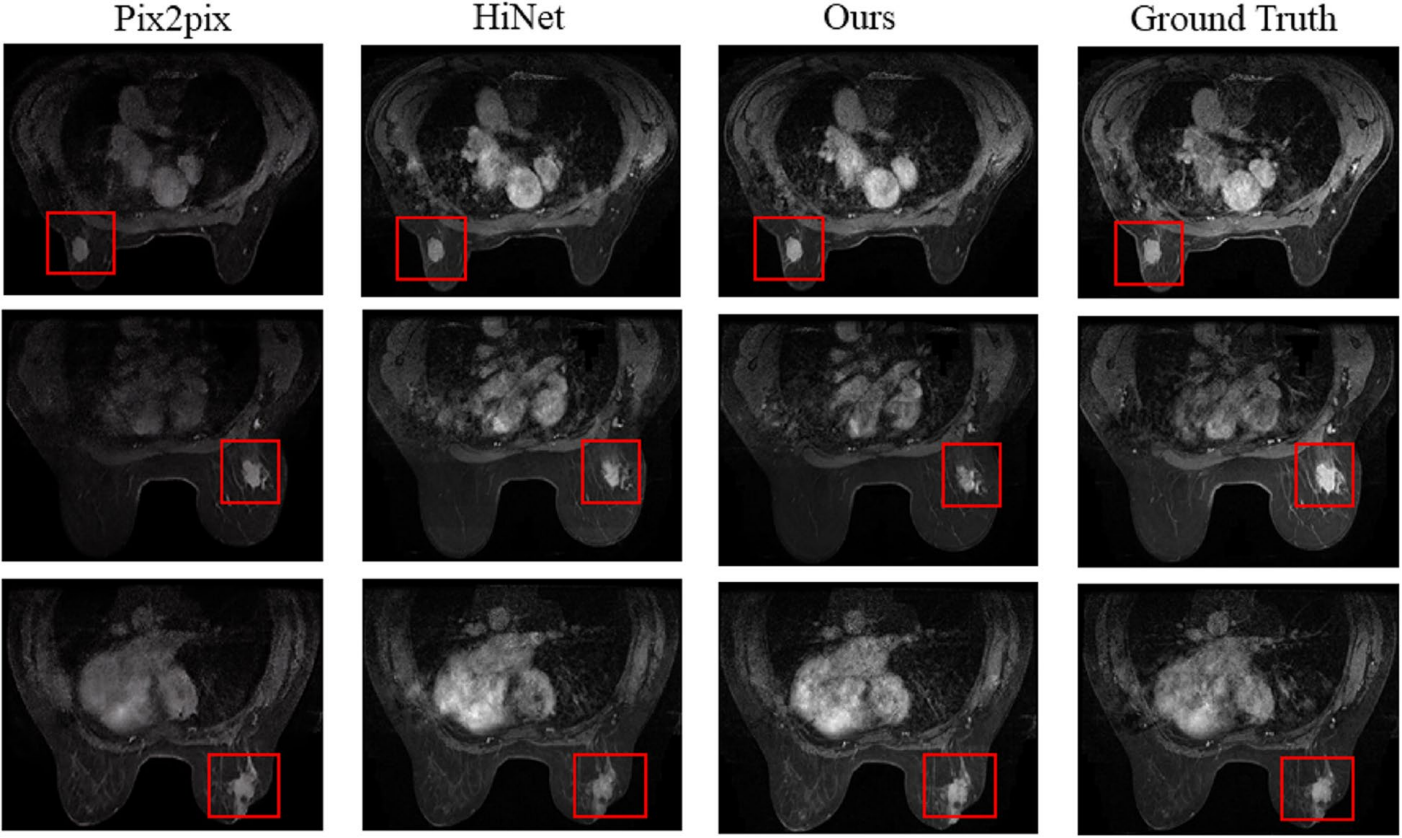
The generated eighth temporal images of DCE-MRI with three methods
